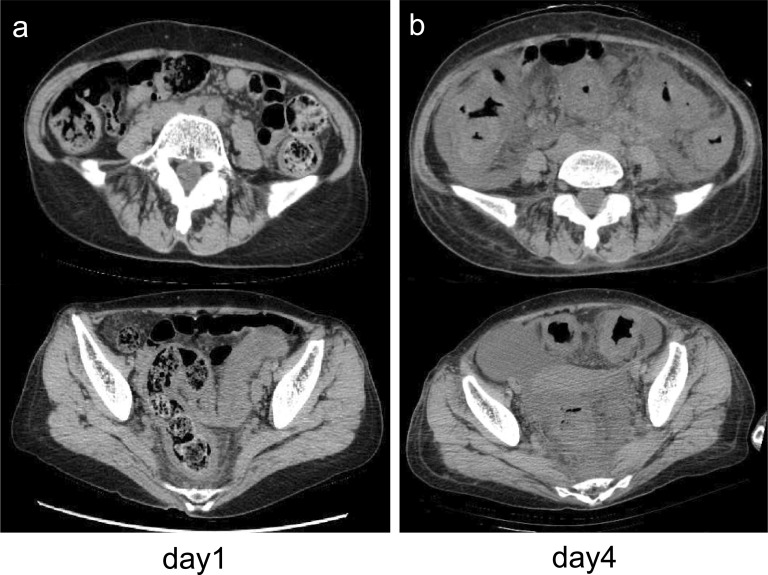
CT shows progressively worsening edematous changes in the intestinal wall and increased ascites from the first (a) to the fourth (b) hospital day
